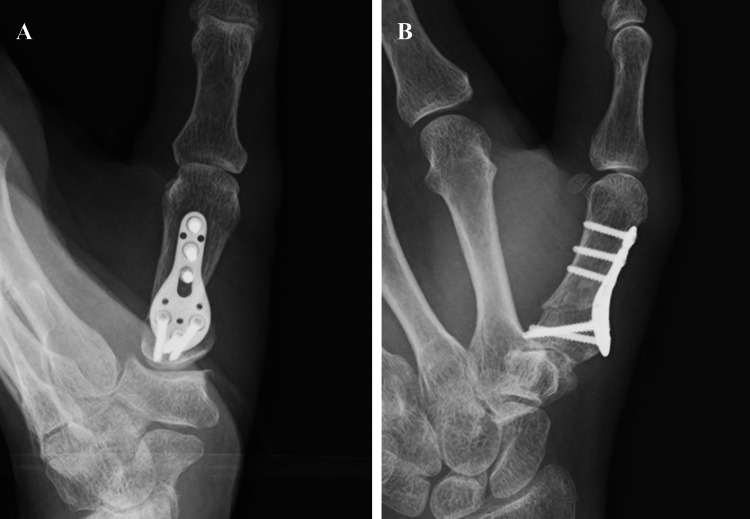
Postoperative radiograph after plate fixation A: Anteroposterior view, B: Lateral view
